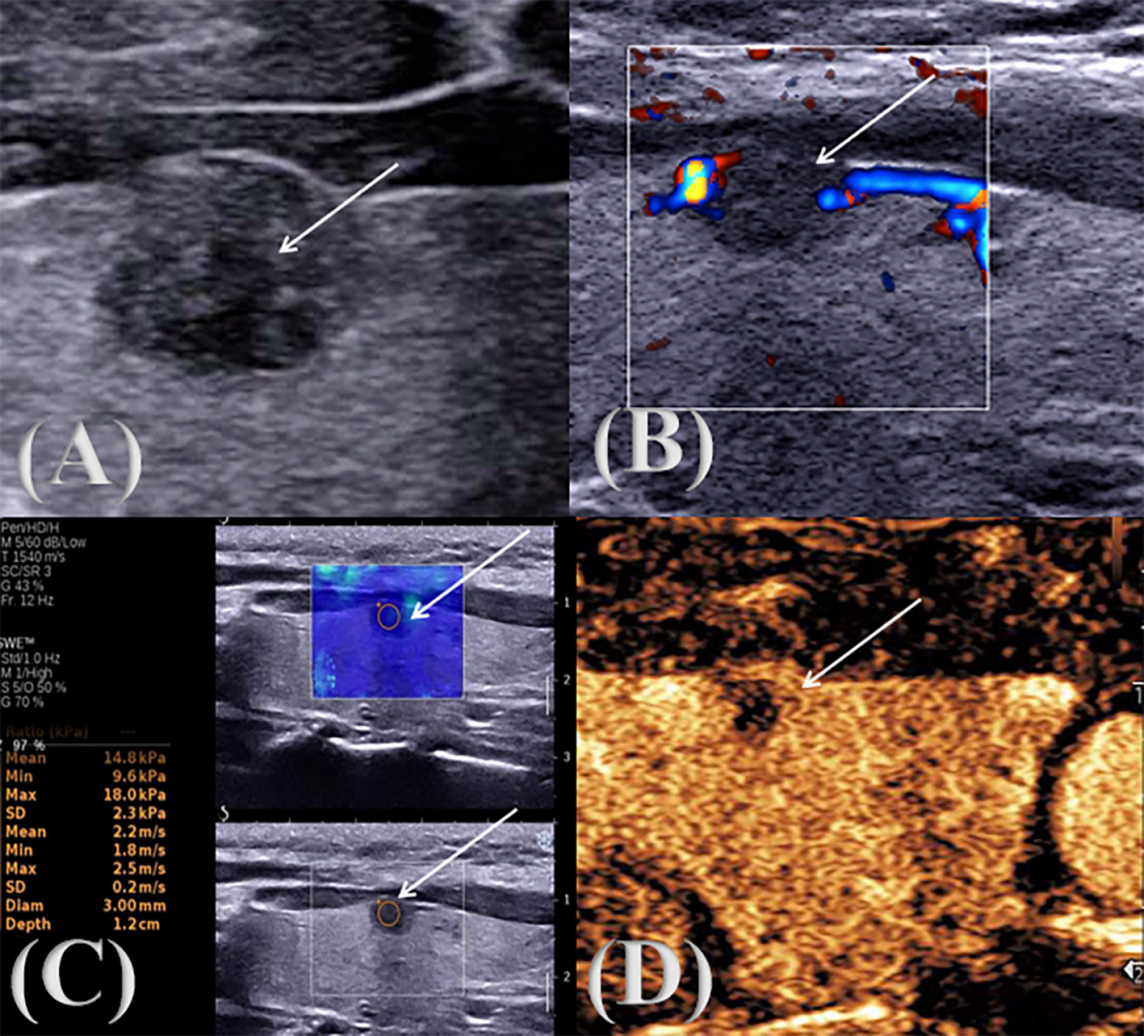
Multimodal ultrasound image of a pathologically confirmed nodular goiter. ↑: Lesion (A) two-dimensional gray scale ultrasonic image; (B) CDFI audio image; (C) SWE image; (D) CEUS audio image.
